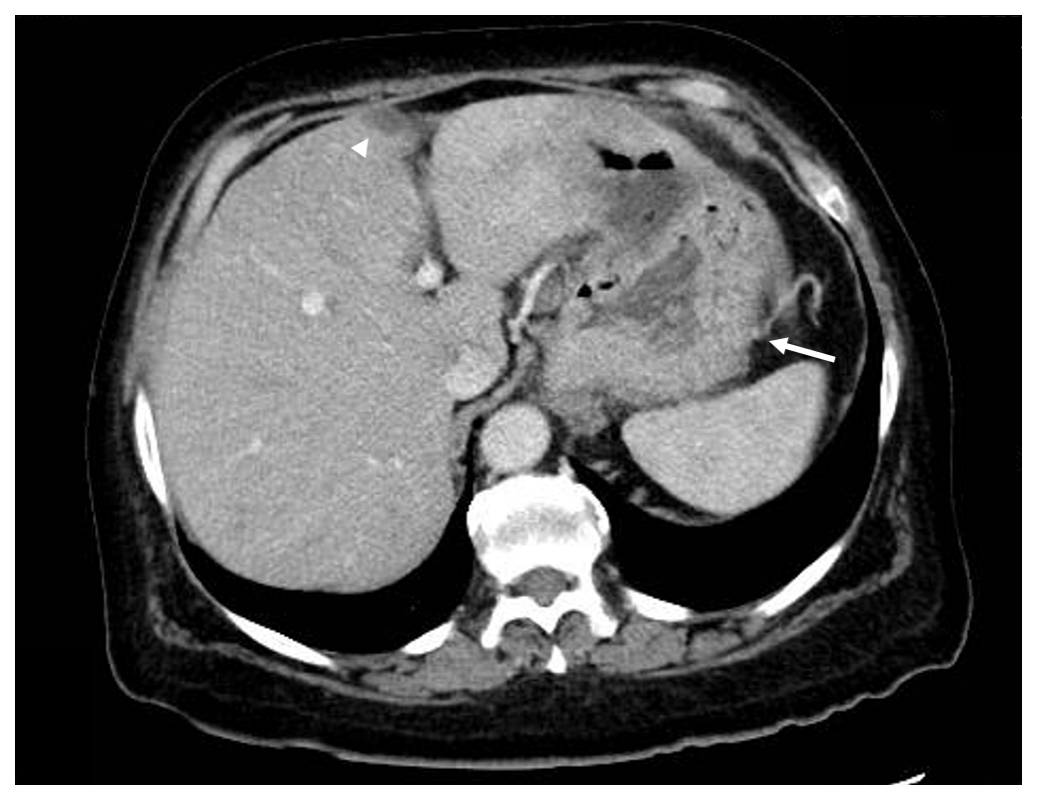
Abdominopelvic CT scan with contrast is primarily nonrevealing for a malignant process. Nonspecific gastric fold thickening in the fundus is observed (
